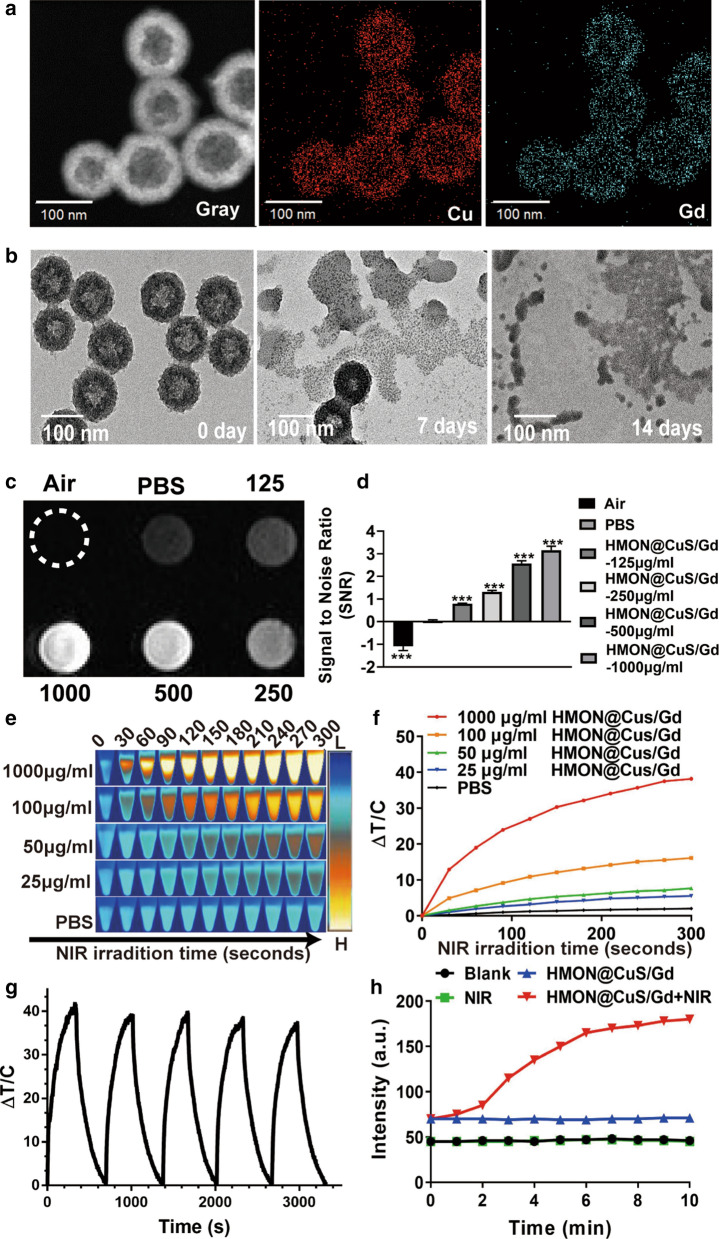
a Elemental mappings of Cu and Gd elements for HMON@CuS/Gd NPs. b TEM images of biodegradable HMON@CuS/Gd NPs immersed in 10 mM GSH aqueous solution for 7 days and 14 days. c, d T1-weighted MRI images of HMON@CuS/Gd at various concentrations (left) and the relative MR signal intensities (right). e, f The temperature increase curve induced by different concentrations of HMON@CuS/Gd aqueous solutions and PBS under NIR irradiation (0.8 W/cm^2^, 5 min). g PTT stability of HMON@CuS/Gd NPs under NIR laser irradiation (0.8 W/cm^2^, 5 min). h The production of singlet oxygen by the HMON@CuS/Gd NPs with or without NIR irradiation (0.8 W/cm^2^, 5 min). Data are shown as mean ± SD, n = 3
